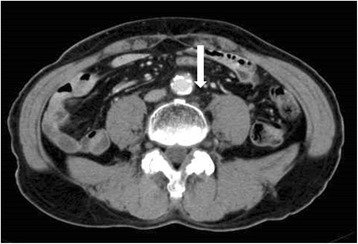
Computed tomography of case 2. An image shows disappearance of the para-aortic lymph metastasis after formalin-fixed tumor vaccine therapy (arrow)
